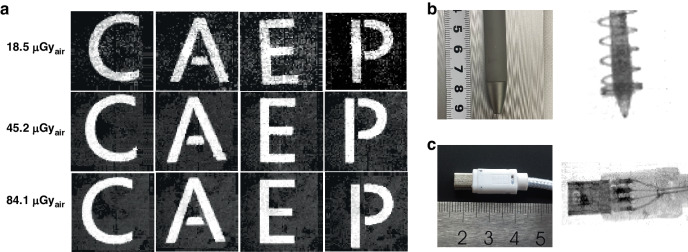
X-ray imaging properities. X-ray images of (a) metal letters at different dose rate, (b) ball-point pen, and (c) data line
